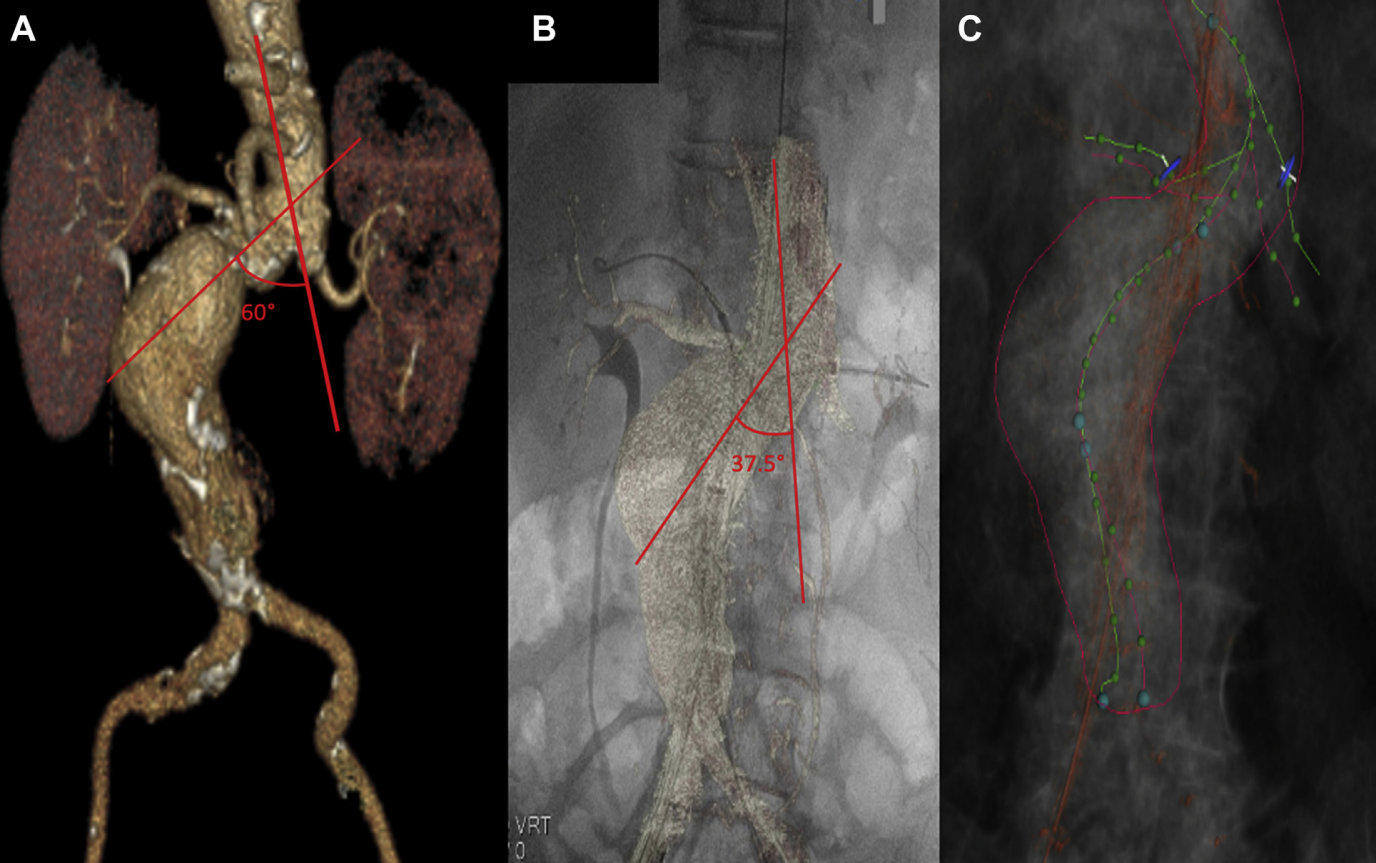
A, Three-dimensional (3D) reconstruction of the preoperative computed tomography (CT) angiogram (CTA). As denoted by the *red lines,* a 60° angle is present at the level of the renal arteries. B, After device introduction and the subtraction spin protocol, we observed a 37.5° angle at the level of the renal arteries. C, Polylines are drawn for the fusion from the preoperative computed tomography (CT) scan (*red polyline*) and from the subtraction spin (*green lines*) to show the differences in the aortic and renal configuration before and after device deployment.
